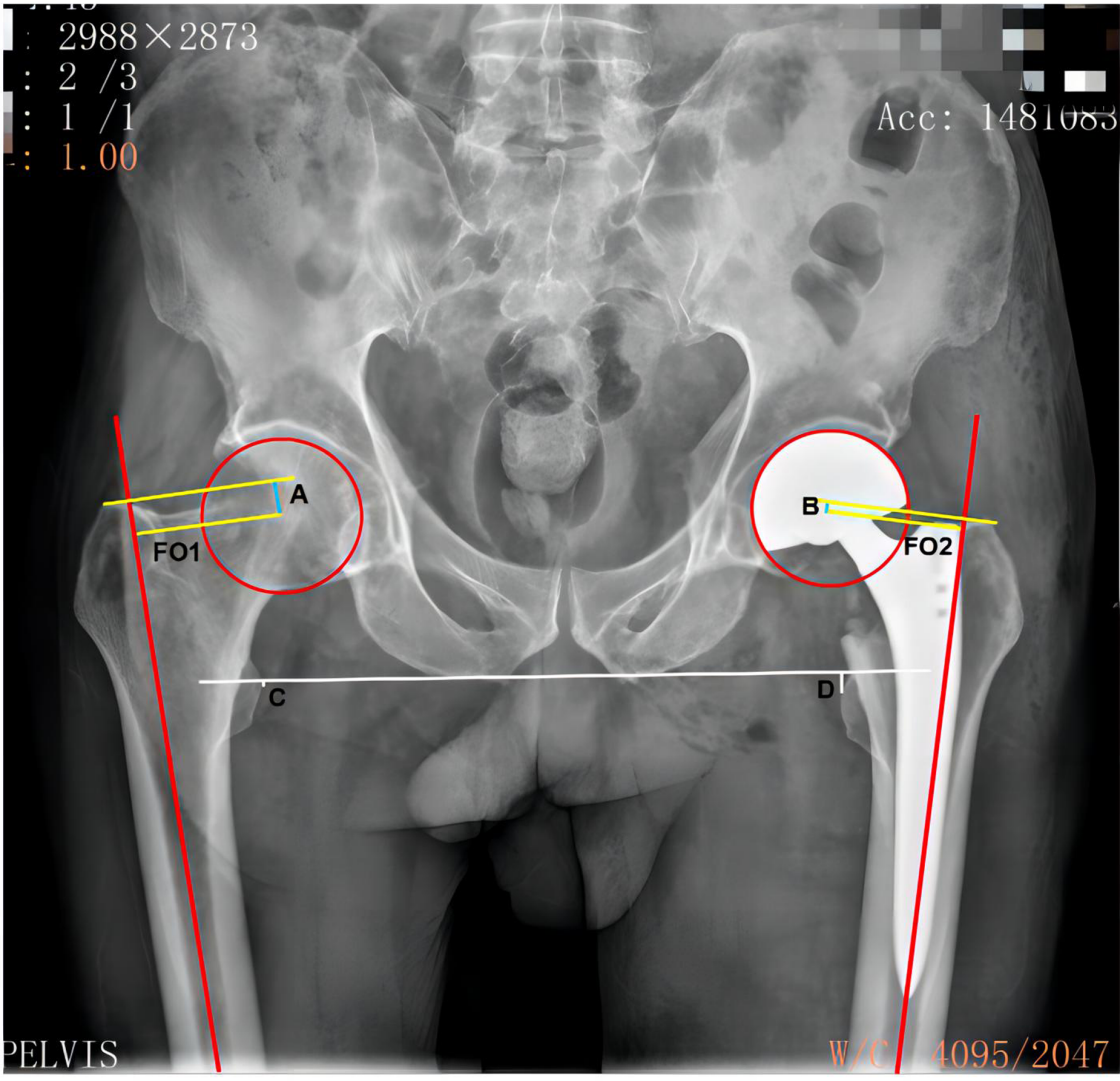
X-ray. (A) the vertical distance from the center of the femoral head to the tip of the greater trochanter on the anatomical axis of the femur on the non-operative side. (B) the vertical distance from the center of the femoral head to the tip of the greater trochanter on the anatomical axis of the femur after surgery on the operative side. (C), the vertical distance from the tip of the lesser trochanter to the connected line of the bilateral sciatic tuberosity after surgery on the non-operative side. (D) the vertical distance from the tip of the lesser trochanter to the connected line of bilateral sciatic tuberosity after surgery on the operative side. The LLD is the difference between the measured values of both sides. FO 1: the distance between the center of rotation of the femoral head and the long axis of the femur on the non-operative side. FO 2: the distance between the center of rotation of the femoral head and the long axis of the femur after surgery on the operative side. The difference between the measured values of the two sides is the FO difference. LLD, leg length discrepancy; FO, femoral offset.
